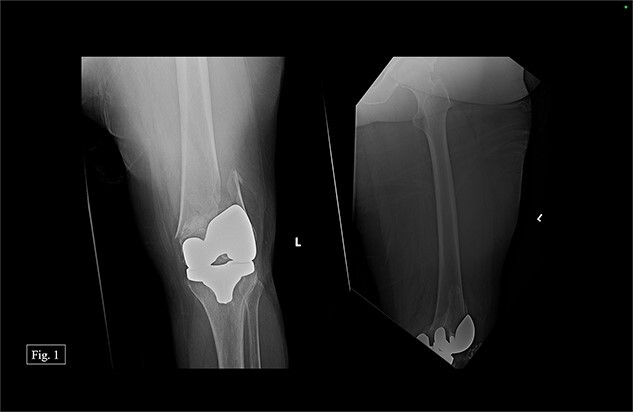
Left, acute distal periprosthetic femur fracture X-ray.
